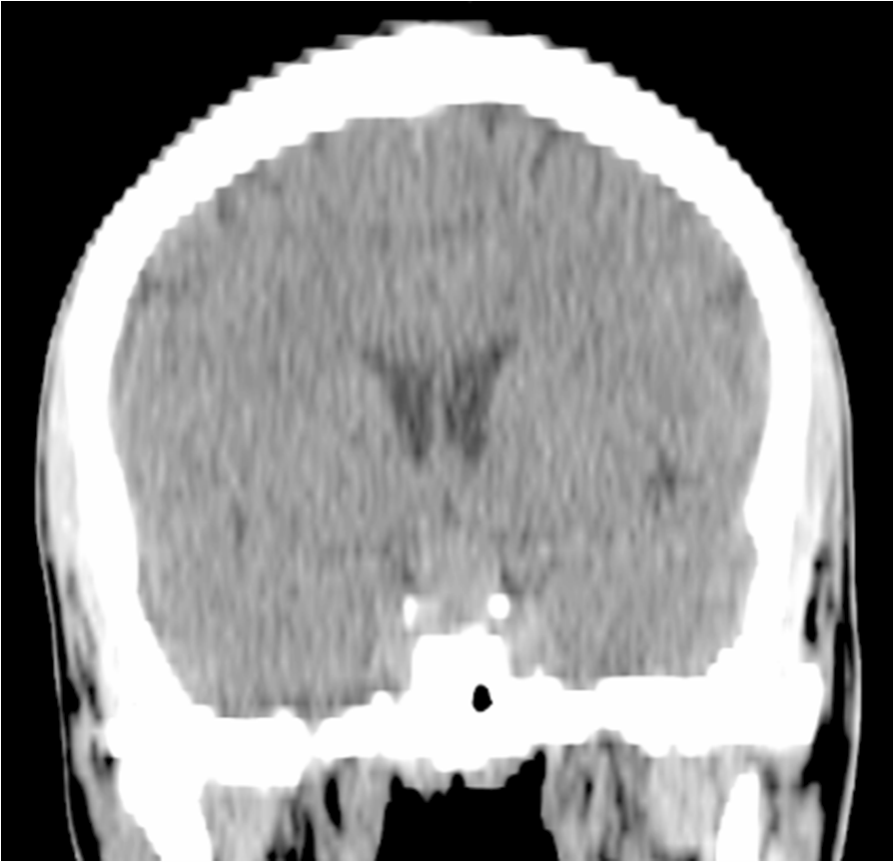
Coronal computed tomography scan section showing a pituitary mass.
